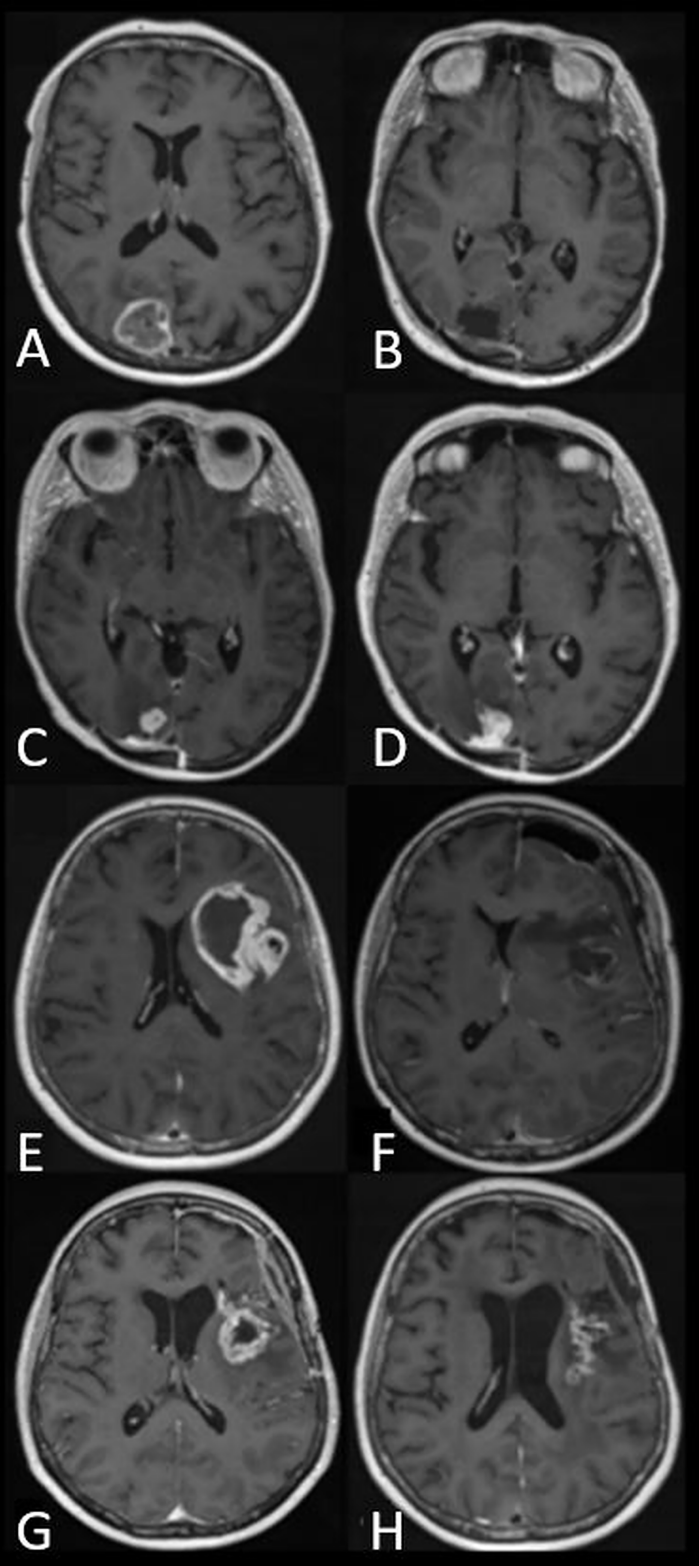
Panels of axial *T*_1_-weighted images after contrast administration in two patients with glioblastoma, IDH-wildtype. (A–D) demonstrate tumor progression. (A) Preoperative appearance of an occipital glioblastoma. (B) MRI performed five days after resection with no enhancement suggestive of residual tumor. (C) The patient continued with standard-of-care radiotherapy and temozolomide. Within three months of radiotherapy, a new enhancing lesion could be seen at the margin of the postoperative cavity. (D) The enhancing lesion had increased in size three months later and was confirmed to represent tumor recurrence after repeat surgery. (E–H) demonstrate pseudoprogression. (E) Preoperative appearance of an insular glioblastoma. (F) Postoperative appearance 24 hours after surgery showing blood degradation products, with no enhancement suggestive of residual tumor. (G) The patient continued with standard-of-care radiotherapy and temozolomide. MRI performed within six months of radiotherapy demonstrated a new, contrast-enhancing lesion. (H) Follow-up MRI at monthly intervals showed a gradual decrease in the size of the contrast-enhancing lesion without a change to standard-of-care temozolomide or corticosteroid use. The image shown here is the MRI performed four months later.
